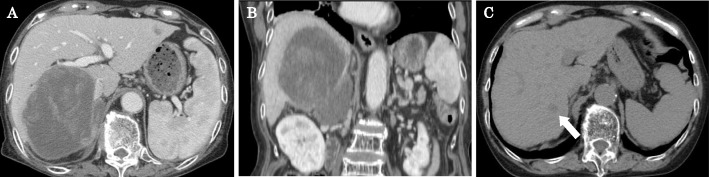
Abdominal contrast-enhanced computed tomography showing a heterogeneous mass lesion in the right lobe of the liver; a axial section, b coronal section. c Four months previously, a 2-cm low-density tumor (arrow) was detected in liver segment 6
